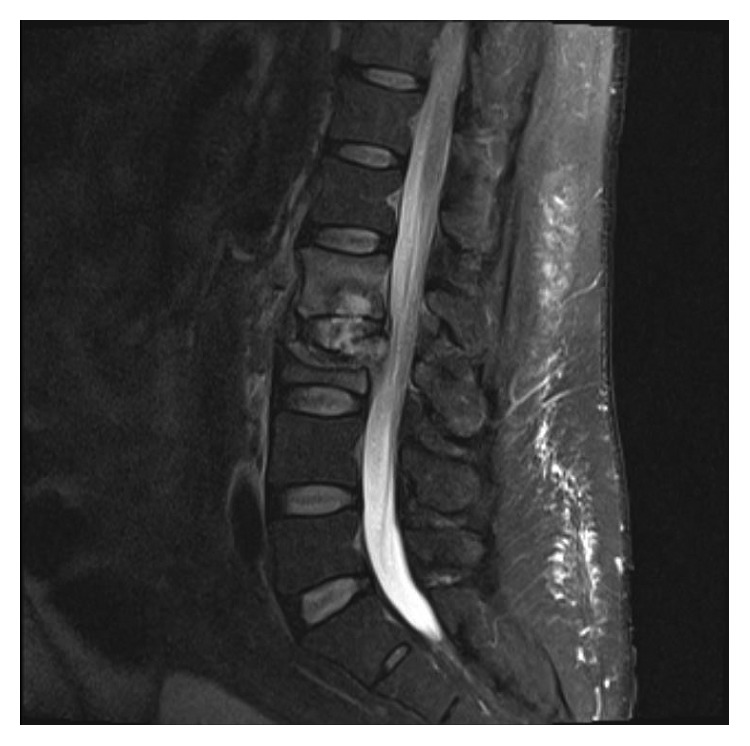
MRI lumbar spine with and without contrast revealing the compression fracture of L3. Ventral epidural enhancement with mass effect on the thecal sac related to an epidural abscess.
